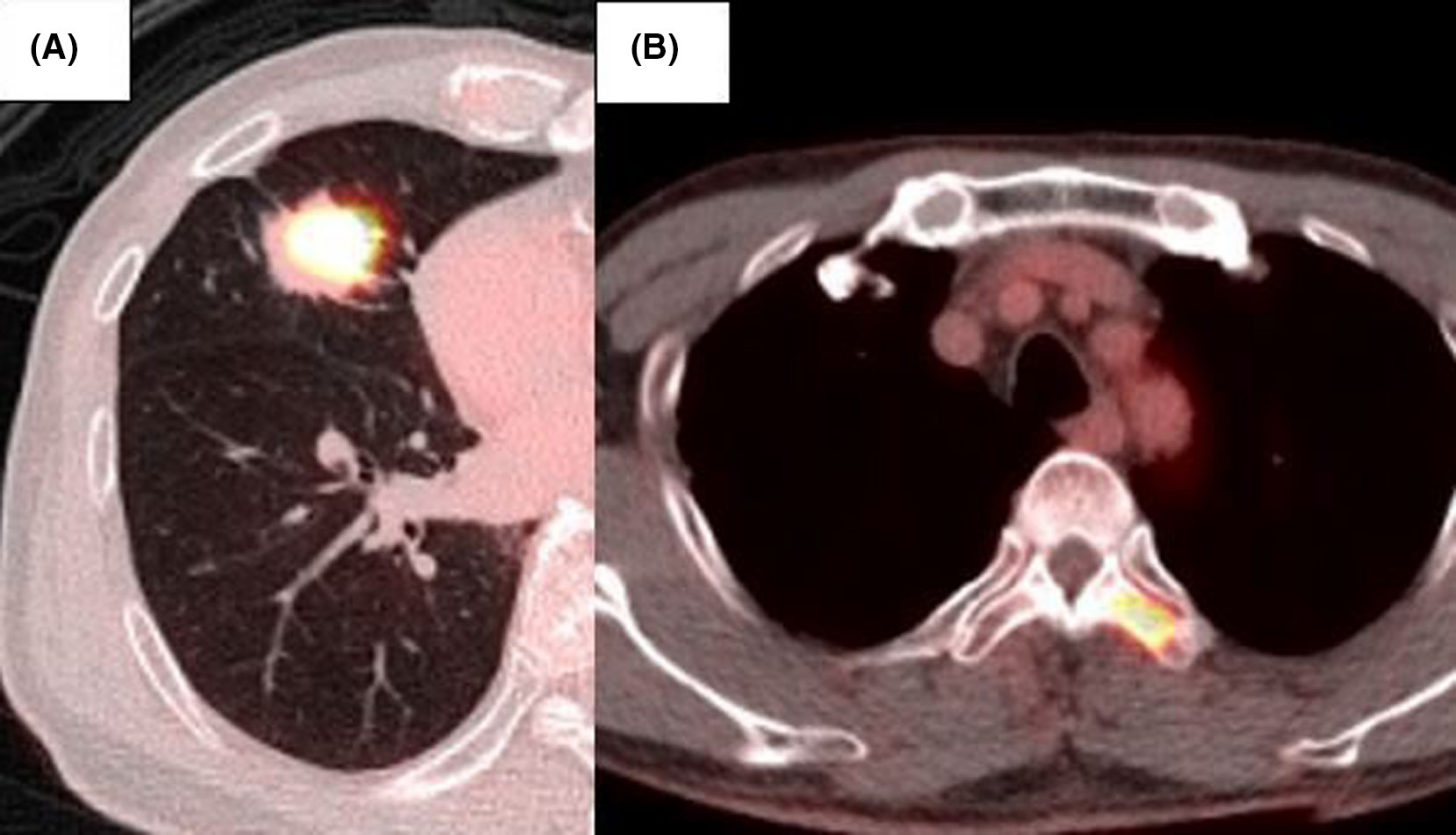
Positron emission tomography surveillance of the mass and discovered bony metastases. (A) Transverse view of the mass with a maximum standardized uptake value (SUVmax) of 7.44. (B) Transverse view at the fourth thoracic vertebra with an SUVmax of 5.32 due to bony metastases of fourth and fifth vertebra
